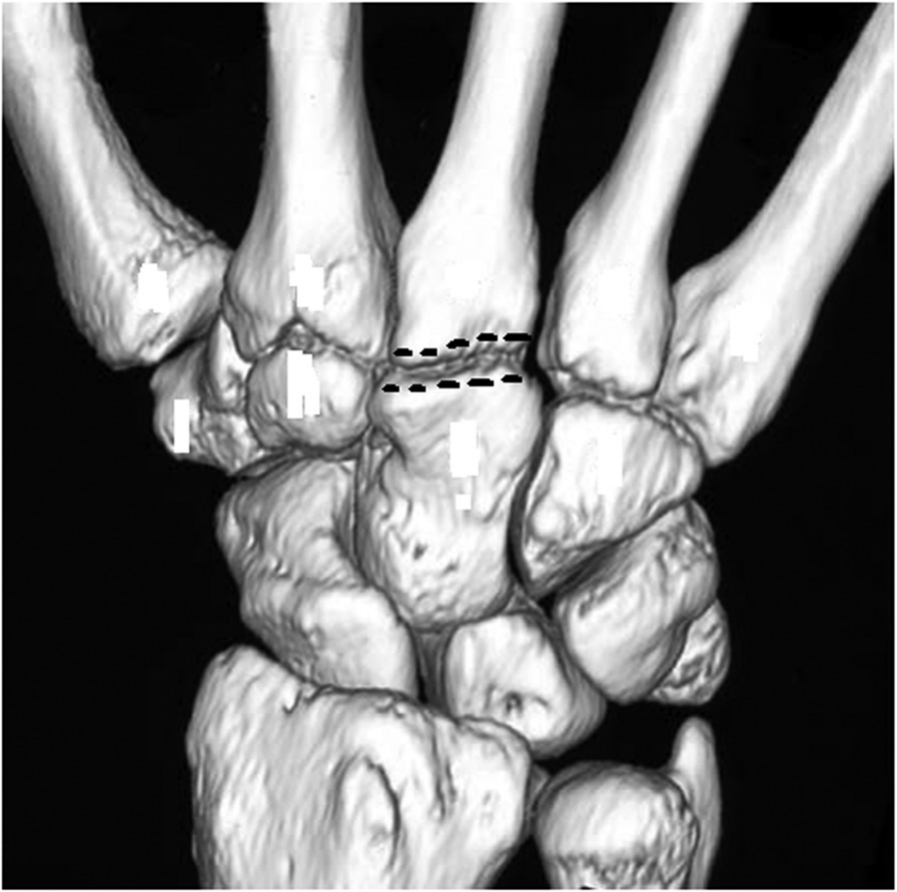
Landmarks on the 3D CT reconstruction of the wrist denoting the site of the saw cuts.
